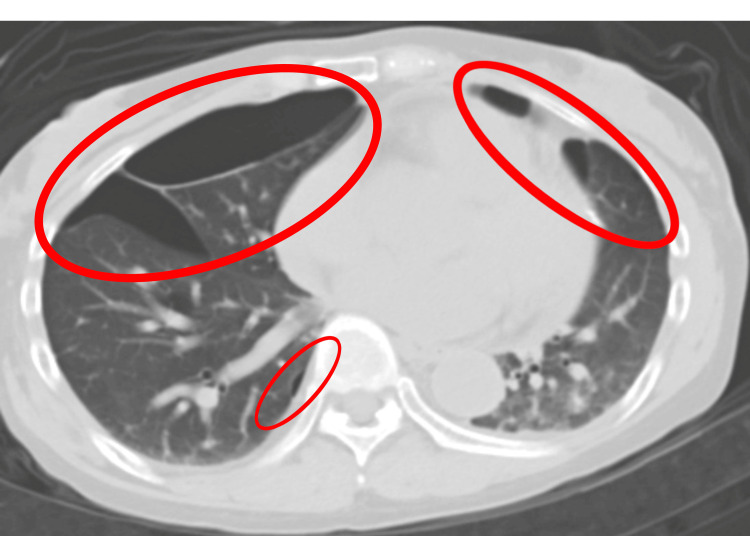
Postoperative chest CT (axial) showing no pneumothorax after third surgery. Postoperative chest CT revealing pulmonary cysts in the same locations (red ovals) as preoperatively, indicating no pneumothorax. CT, Computed Tomography
